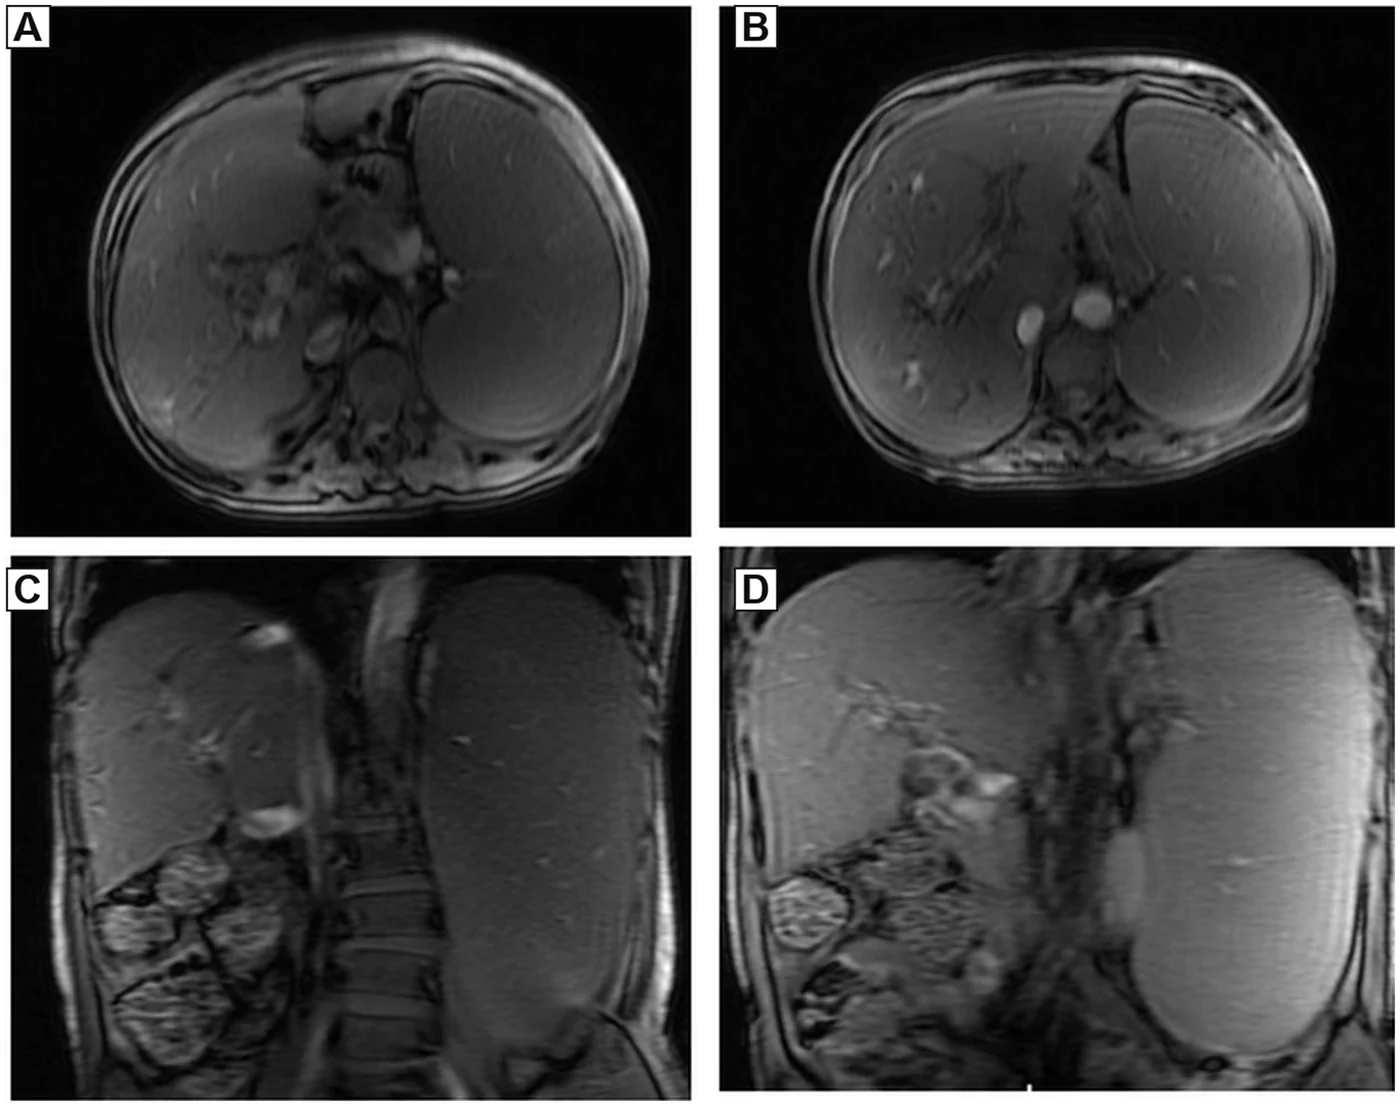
Upper abdominal MRI/MRCP showing portal-hypertensive changes. (A,B) Axial and (C,D) coronal images demonstrate marked splenomegaly, poor visualization of the main portal vein and its right and left branches, and cavernous transformation of the portal vein.
